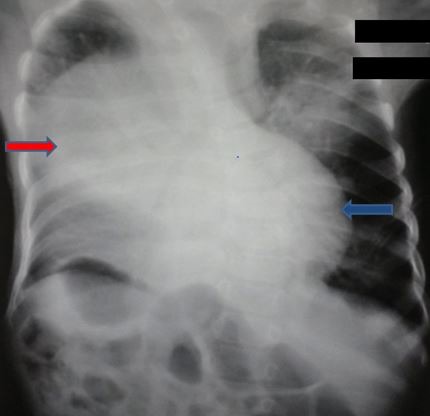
radiographie de thorax face: opacité médiastinale antérieure (flèche rouge) effaçant les bords du cœur (flèche bleu), élargissant le médiastin moyen et inférieur
